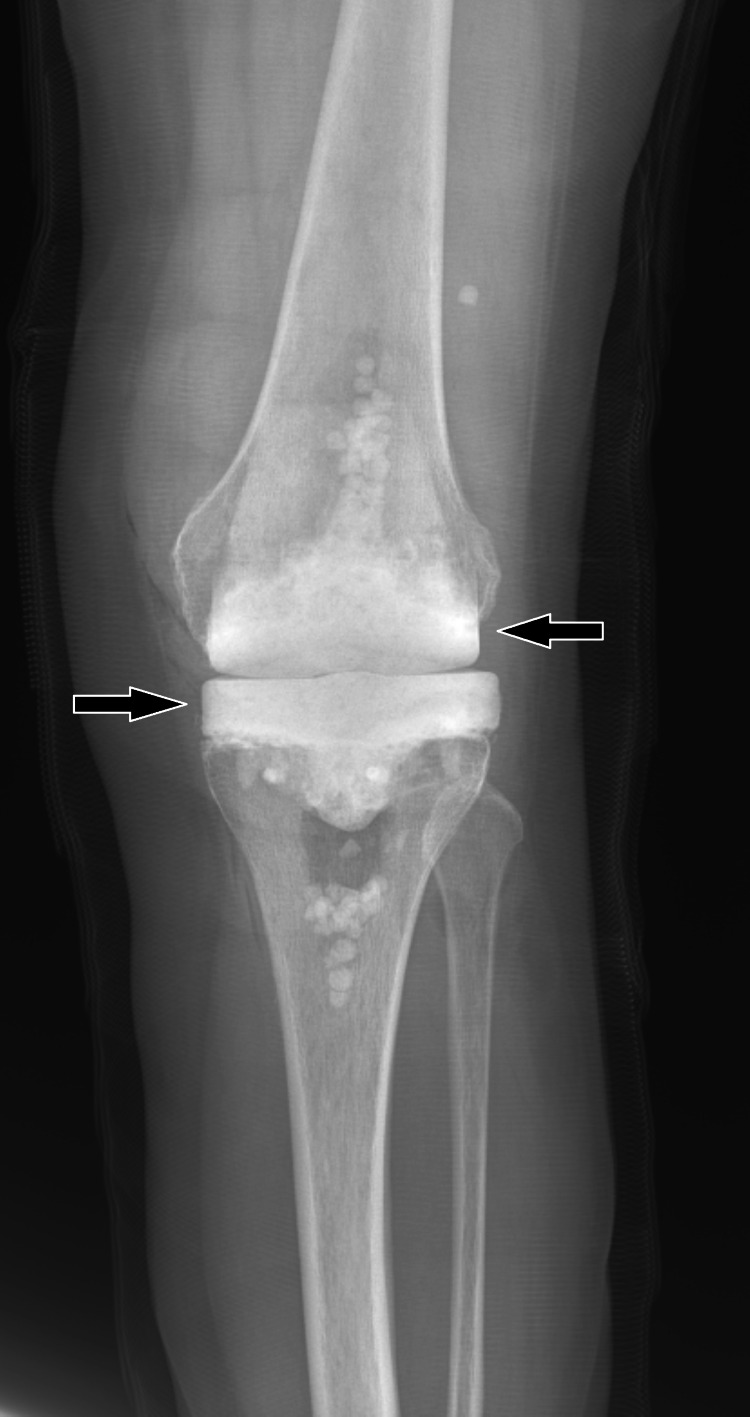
Left knee X-ray showing femoral and tibial spacers created from Cobalt Bone Cement with Gentamicin (black arrows).
